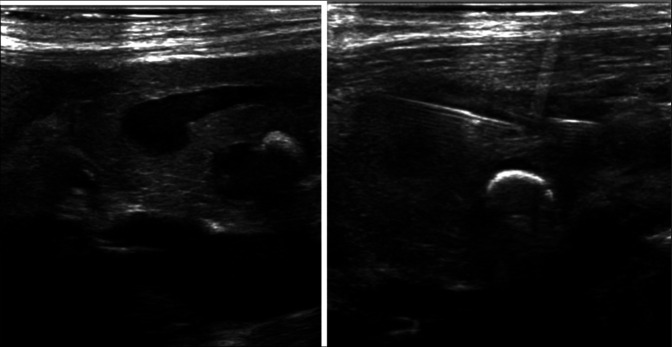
The dilated pancreatic duct was accessed via a direct percutaneous puncture under ultrasound guidance. A large shadowing stone can be seen in the dilated uncinate duct.
